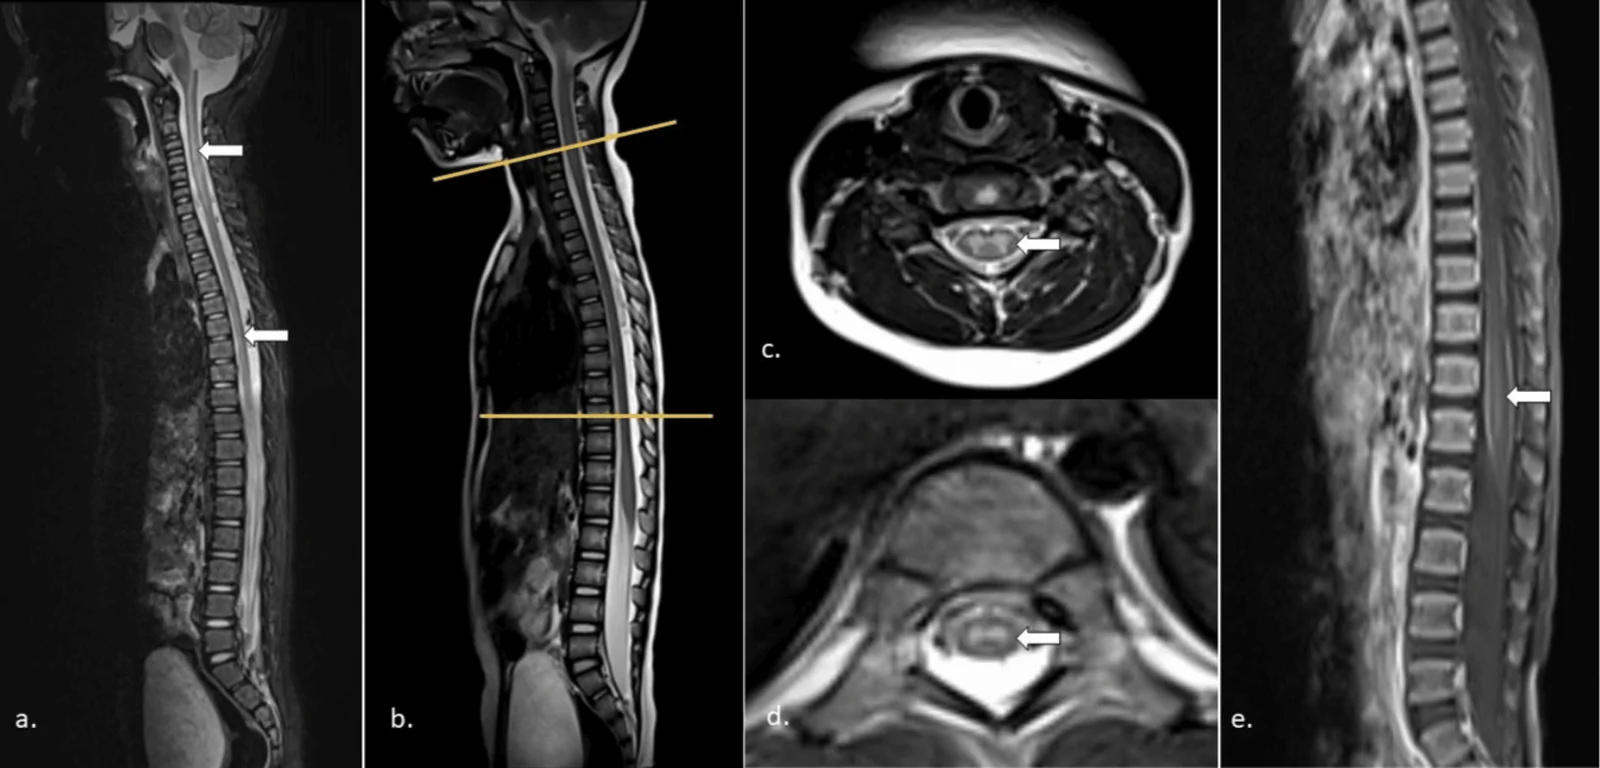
Magnetic resonance imaging of the spine. (a) Whole-spine short-TI inversion recovery (STIR) sagittal image showing STIR hyperintensity in the cervicodorsal spinal cord (arrows). (b) Whole-spine T2-weighted sagittal image showing subtle T2 hyperintensity in the cervicodorsal spinal cord. (c and d) Axial T2-weighted images at the cervical and dorsal spinal cord, respectively, showing central grey-matter hyperintensity (arrows). (e) Post-contrast sagittal dorsolumbar cord image showing post-contrast enhancement involving the conus (arrow).
